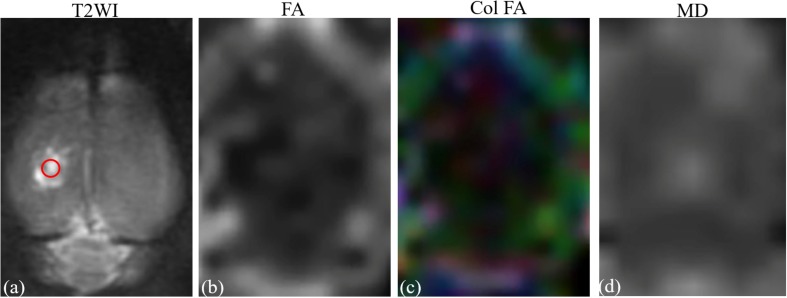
(a) T2-weighted image. (b) Fractional anisotropy map. (c) Color fractional anisotropy map. (d) Mean diffusion map. ROI of injury area (red circle in A) was chosen on axial T2-weighted image with which the fractional anisotropy map and T2-weighted image co-registered.
